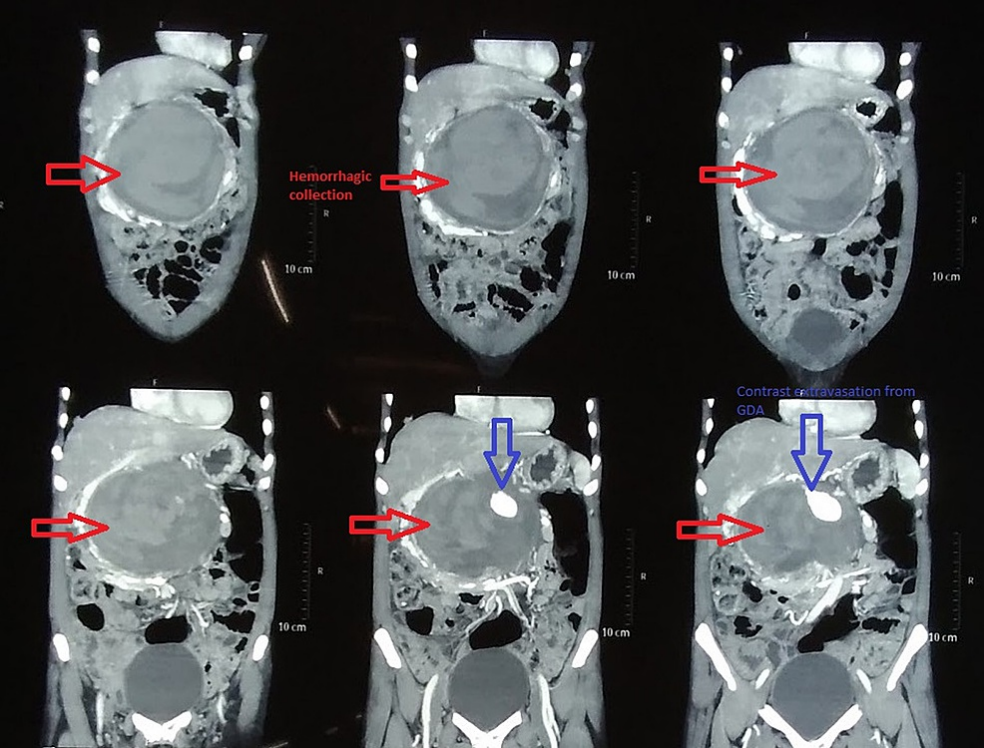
CT: Coronal sections showing retroperitoneal hemorrhagic collection. CT: computed tomography; GDA: gastroduodenal artery
